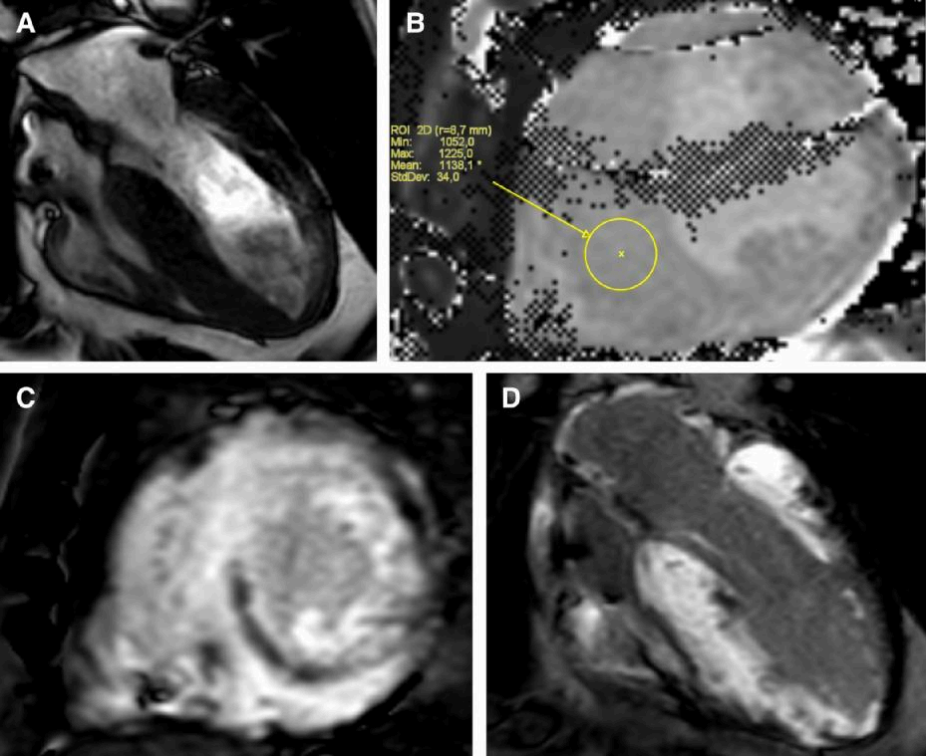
Repeat cardiac MRI performed following recurrent episodes of ventricular tachycardia (2023). (*A*) Cardiac MRI demonstrating worsening of the patient’s left ventricular hypertrophy (interventricular septum newly hypertrophied to 25 mm) and further reduction in LVEF to 26%. Notably, hypertrophy of the interatrial septum is also seen. (*B*) Elevation in the patient’s native T1 signal. (*C*, *D*) Diffuse, circumferential late gadolinium enhancement of all four cardiac chambers appreciated in 2023, which notably includes the atria and lateral wall of the right ventricle. The presence of sub-endocardial sparing argues against an ischaemic aetiology.
